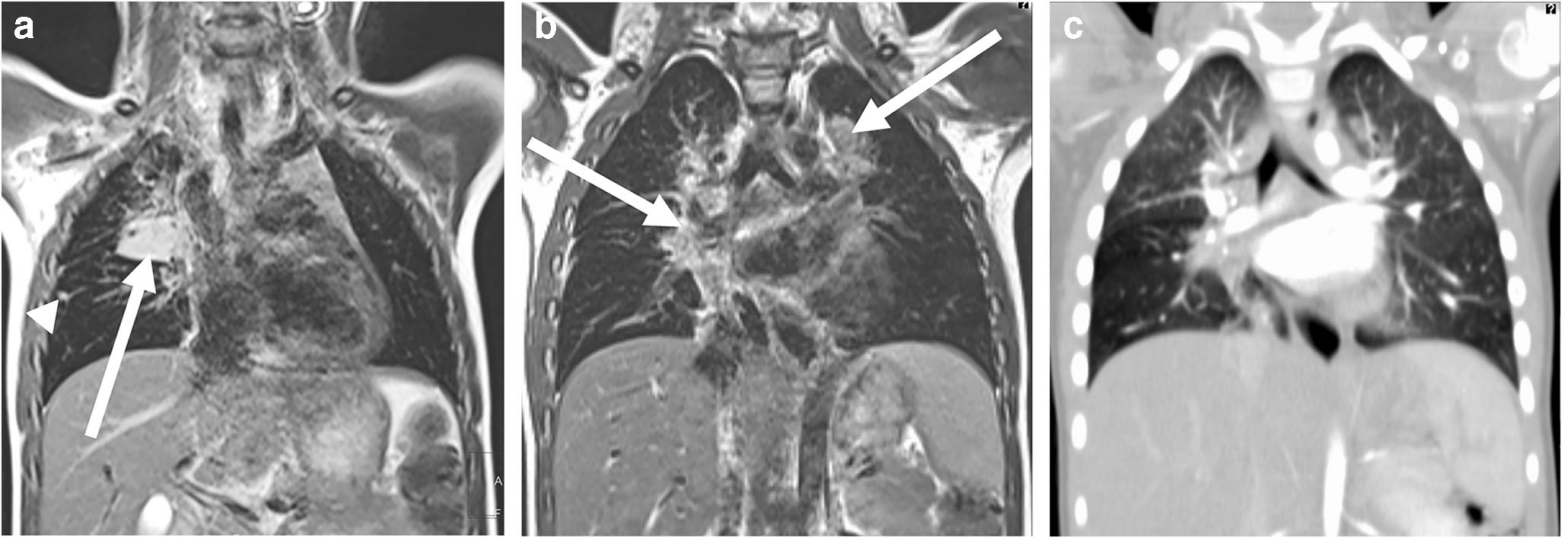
MRI of lung tuberculosis in a 1-year-old boy (MR parameters, Table 2). a Coronal T2-weighted turbo spin-echo image shows an infiltrate (*arrow*) in the right upper lobe. Note also the 3-mm nodular infiltrates (*arrowhead*) in the right lung. b Coronal T2-weighted turbo spin-echo image shows swelling of hilar lymph nodes (*arrows*) and evidence of central peribronchial infiltrations. c Coronal reformat of a low-dose contrast-enhanced CT for comparison
